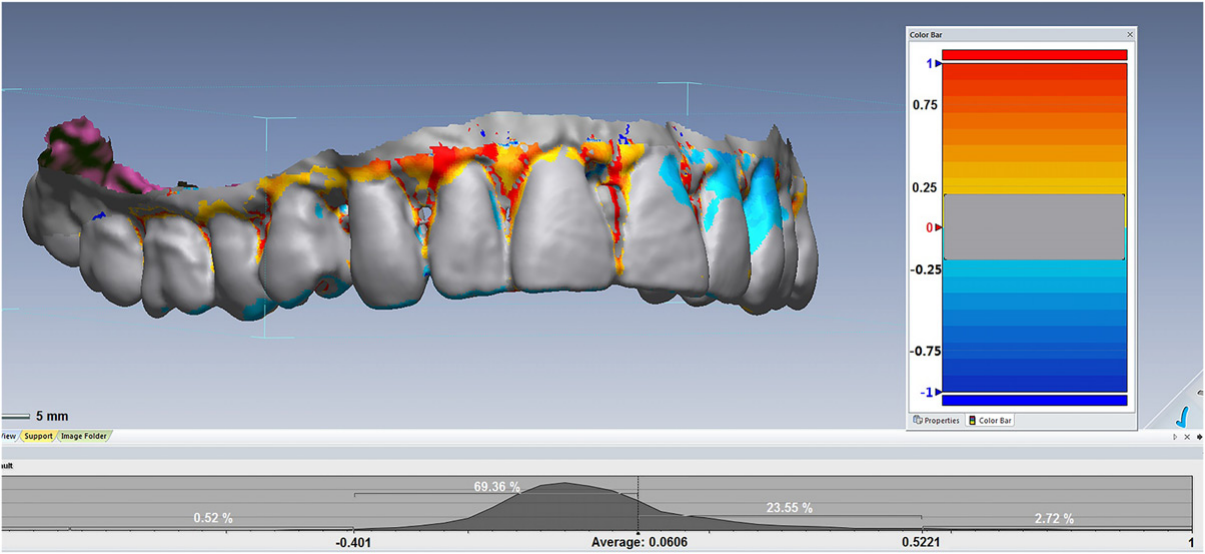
Three-dimensional (3 D) superimposition. The postmortem (PM) surface is superimposed on the antemortem (AM) surface. In this case, scans were secured by a low-resolution medical CT scanner with an approximately 0.3 slice thickness. Surfaces matching within a region of 0.5 mm are coloured grey, while those that fall outside this range (mainly soft tissues) are coloured according to the degree of mismatch. With modern intraoral scanners, the resolution and scan precision are much higher, so the width of the grey zone can be reduced to a level measured in tens of microns, providing excellent individuation. (Image courtesy Ms Donna MacGregor and Mr Mark Barry, with permission).
